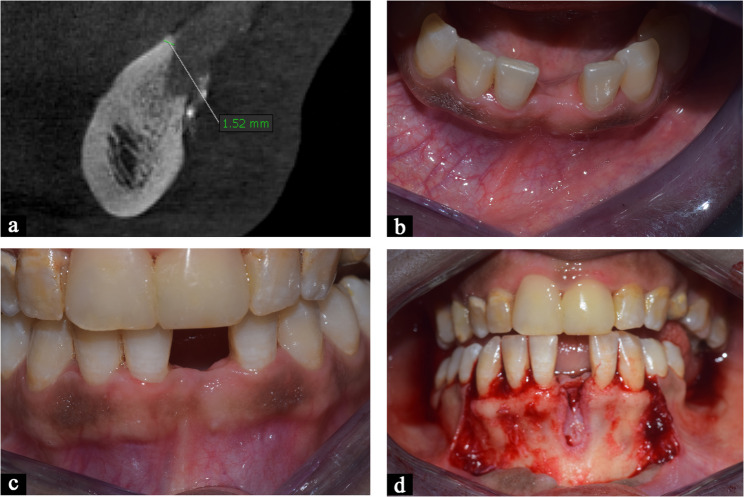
a Preoperative CBCT from DVR module; note radiopaque artefact from previous failed root canal treatment b Preoperative situation occlusal view c Preoperative situation frontal view d Flap elevation
